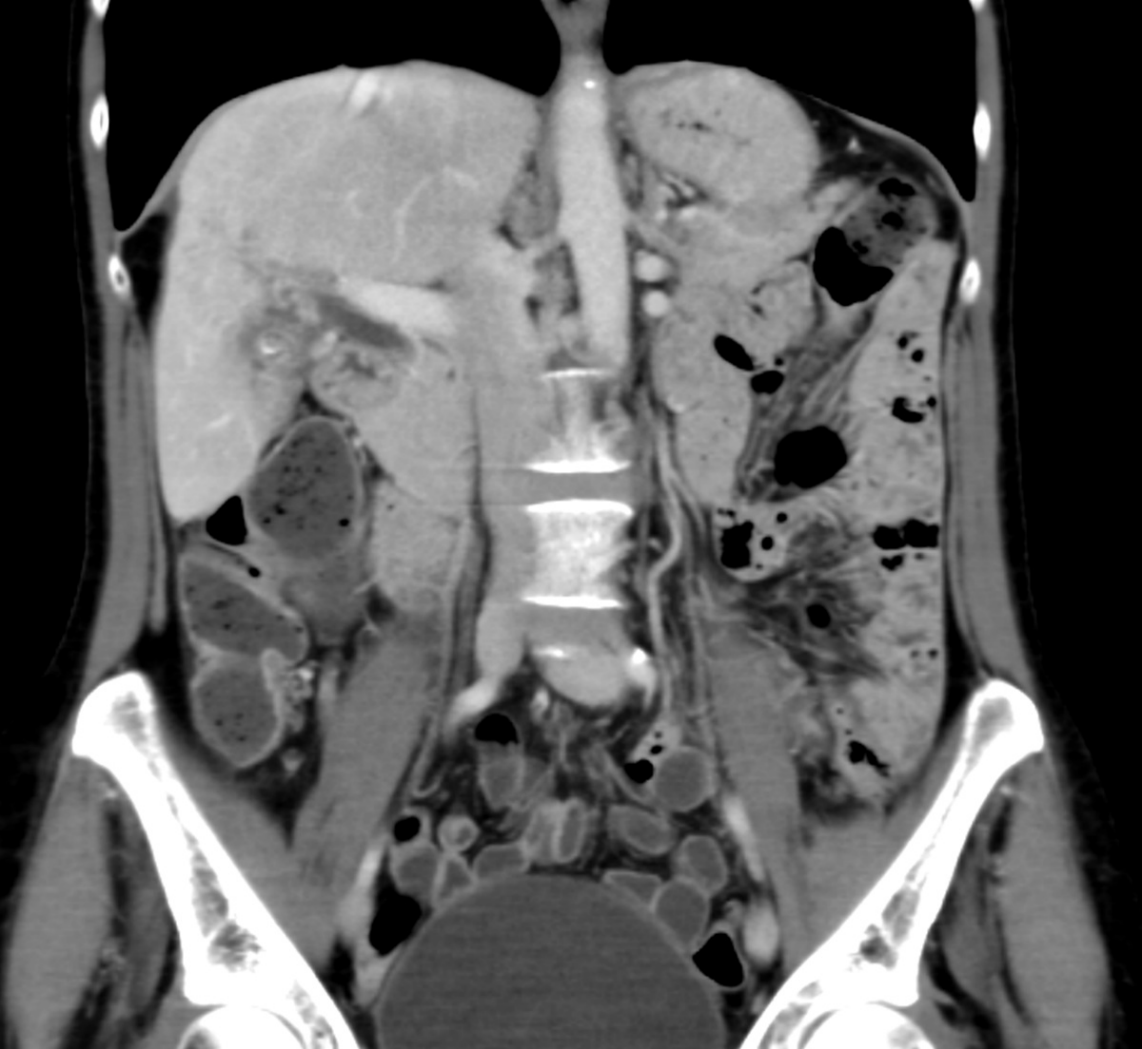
Representative coronal slice from CT obtained nine months after second SIRT procedure
